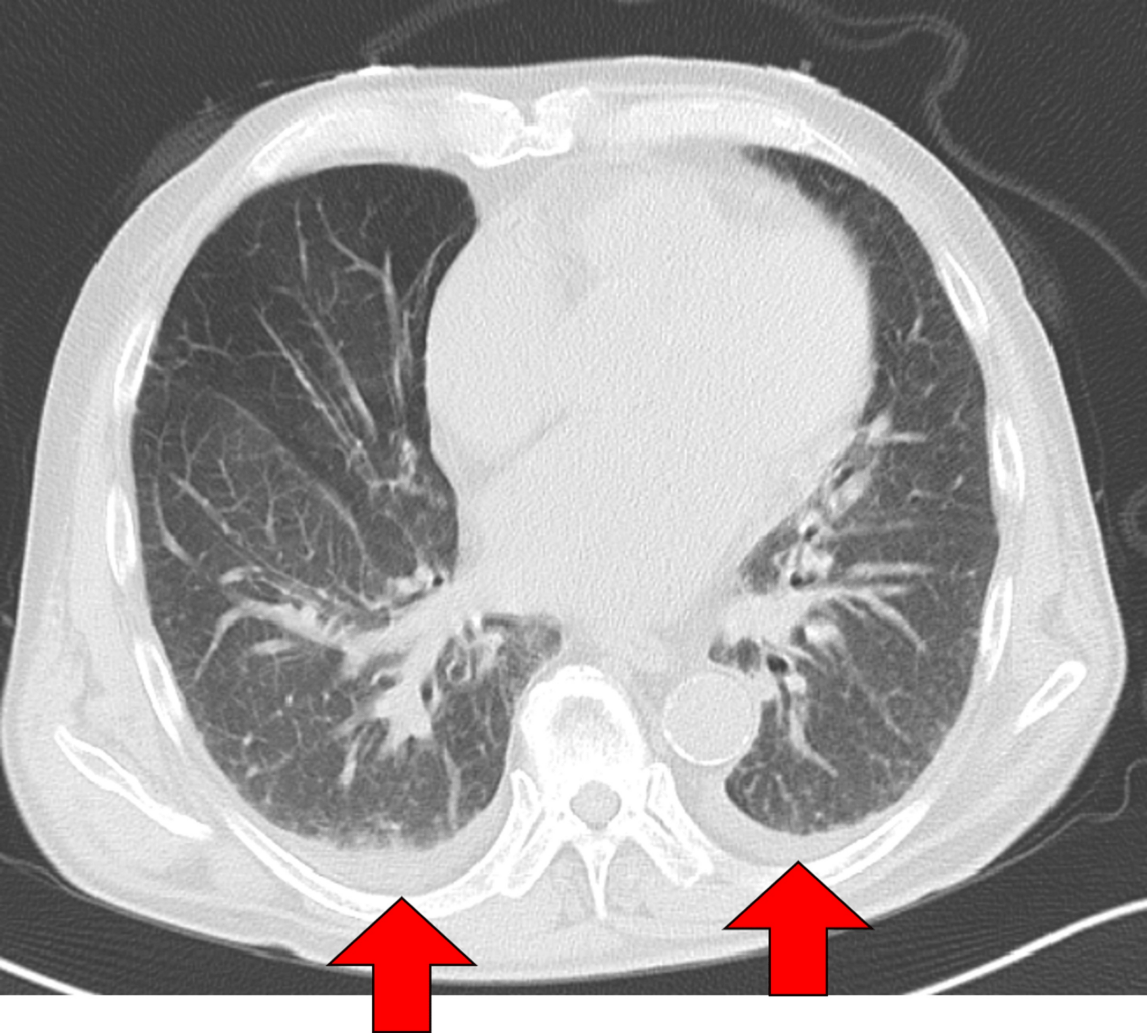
Chest CT on admission to our hospital showing pleural effusion (red arrow).
